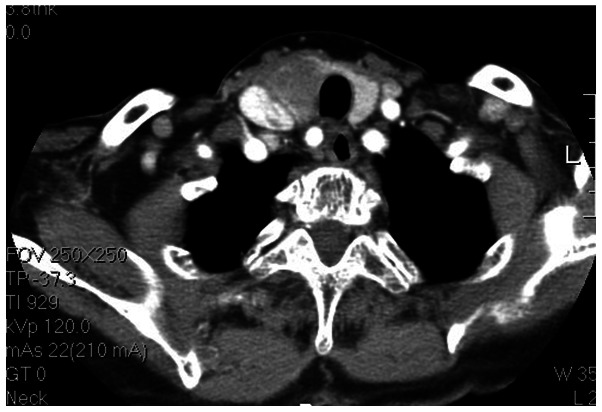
Cervical computed tomography revealed a 3×3×6-cm solid mass in the right thyroid.
